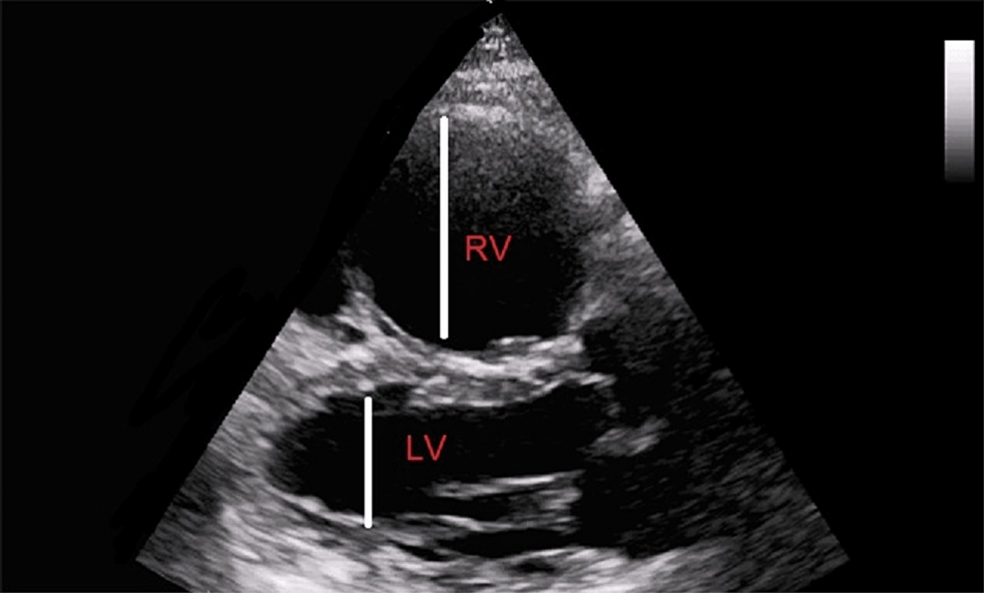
Bedside ultrasound showing dilated RV in comparison to LV RV: Right ventricle; LV: Left ventricle.
